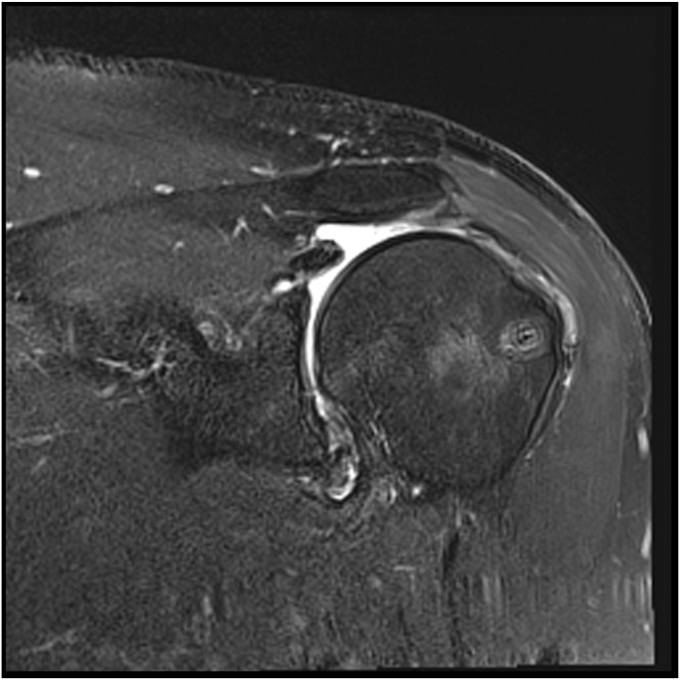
Failed rotator cuff repair at the musculotendinous junction of patient 18. MRI performed 8 months after surgery.
